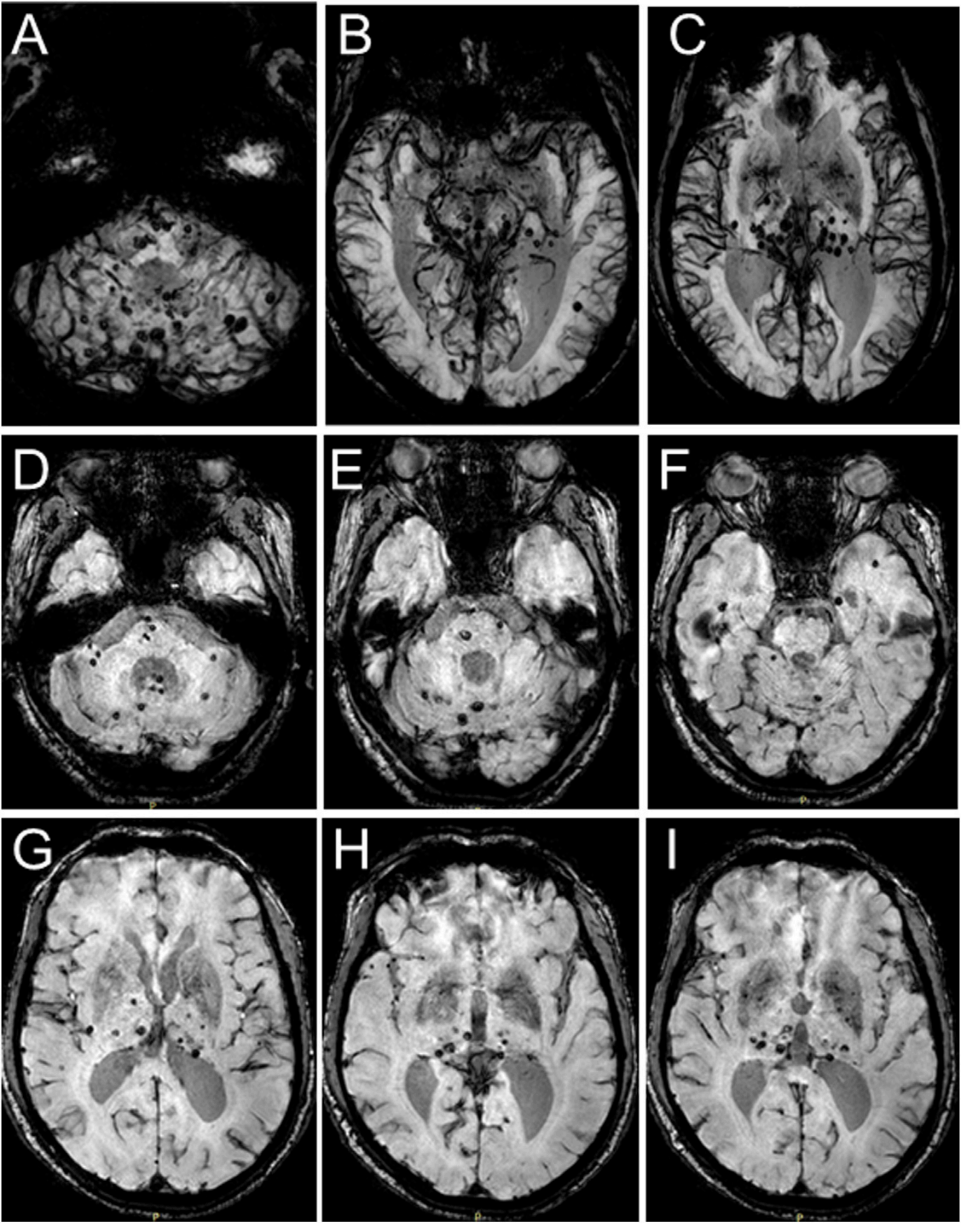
Brain MRI-SWI images of the proband. SWI images present with multiple hypointense signal intensities at several brain levels and demonstrate multiple foci of hemorrhage in the proband’s brain.
